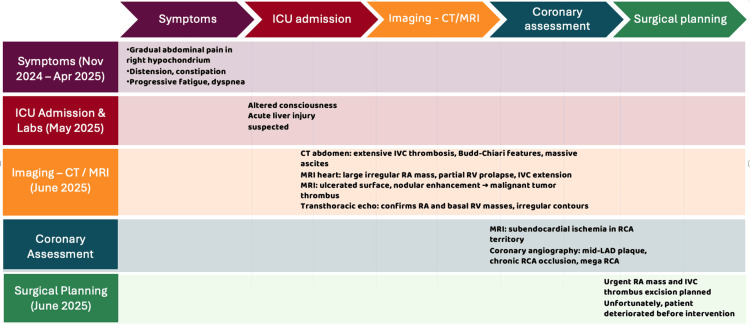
Timeline: Clinical presentation, labs, and imaging from symptom onset to ICU admission. Image credit: Timeline created by the authors from anonymized clinical data (original work). MRI, magnetic resonance imaging; ICU, intensive care unit
